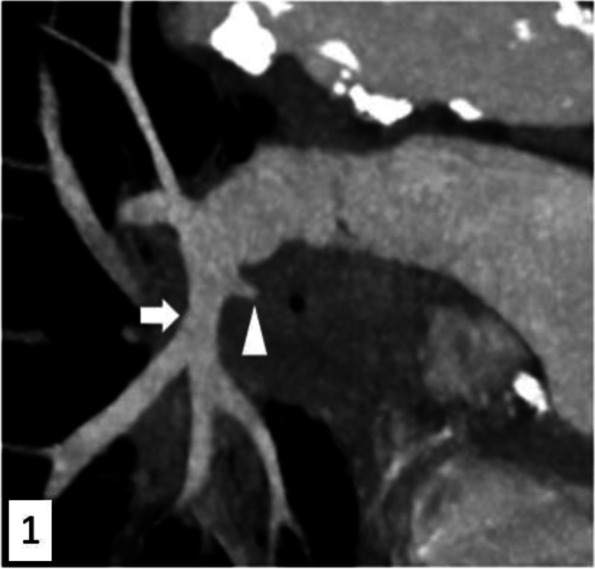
Treatment of a ruptured pulmonary artery pseudoaneurysm with a stent graft. Sagittal reconstruction of a computed tomography angiography before embolization shows a pseudoaneurysm (*arrow head*) arising from the left inferior pulmonary artery root (*arrow*). Sagittal MIP 10 mm reconstruction 80 keV 130 mAs Somatom X.Cite Siemens Healthineers Erlangen
